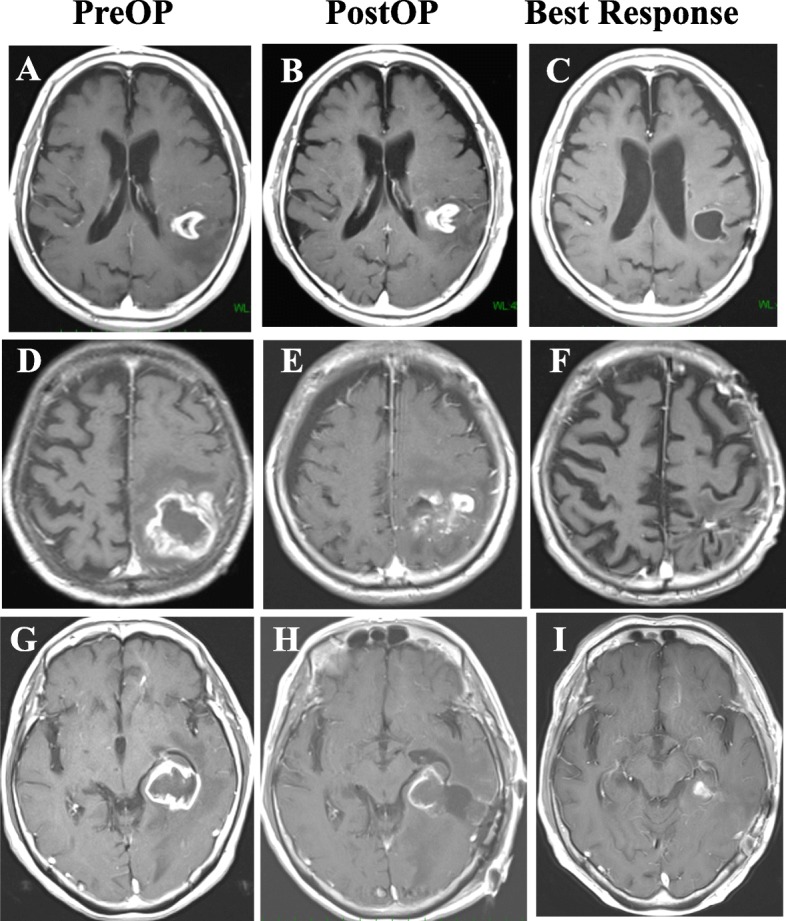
Three representative patients whose T1-weighted magnetic resonance images with gadolinium enhancement showed a partial response. a An 80-year-old man presented with a left temporo-parietal enhanced tumor and underwent biopsy. b Afterwards, he received temozolomide combined with radiotherapy consisting of 45 Gy in 15 fractions and showed a remarkable response with cystic degeneration. c The tumor was *MGMT* promoter-hypomethylated. d A 78-year-old man presented with a left parietal enhanced tumor and underwent partial resection. e Afterwards, he received temozolomide combined with radiotherapy consisting of 45 Gy in 15 fractions and nearly achieved a complete response. f The tumor was *MGMT* promoter-hypermethylated. g A 77-year-old woman presented with a left temporal enhanced tumor and underwent partial resection. h Afterwards, she received temozolomide combined with radiotherapy consisting of 45 Gy in 15 fractions and achieved tumor size reduction. i The tumor was *MGMT* promoter-hypomethylated. Abbreviations: *MGMT* O-6-methylguanine DNA methyltransferase
